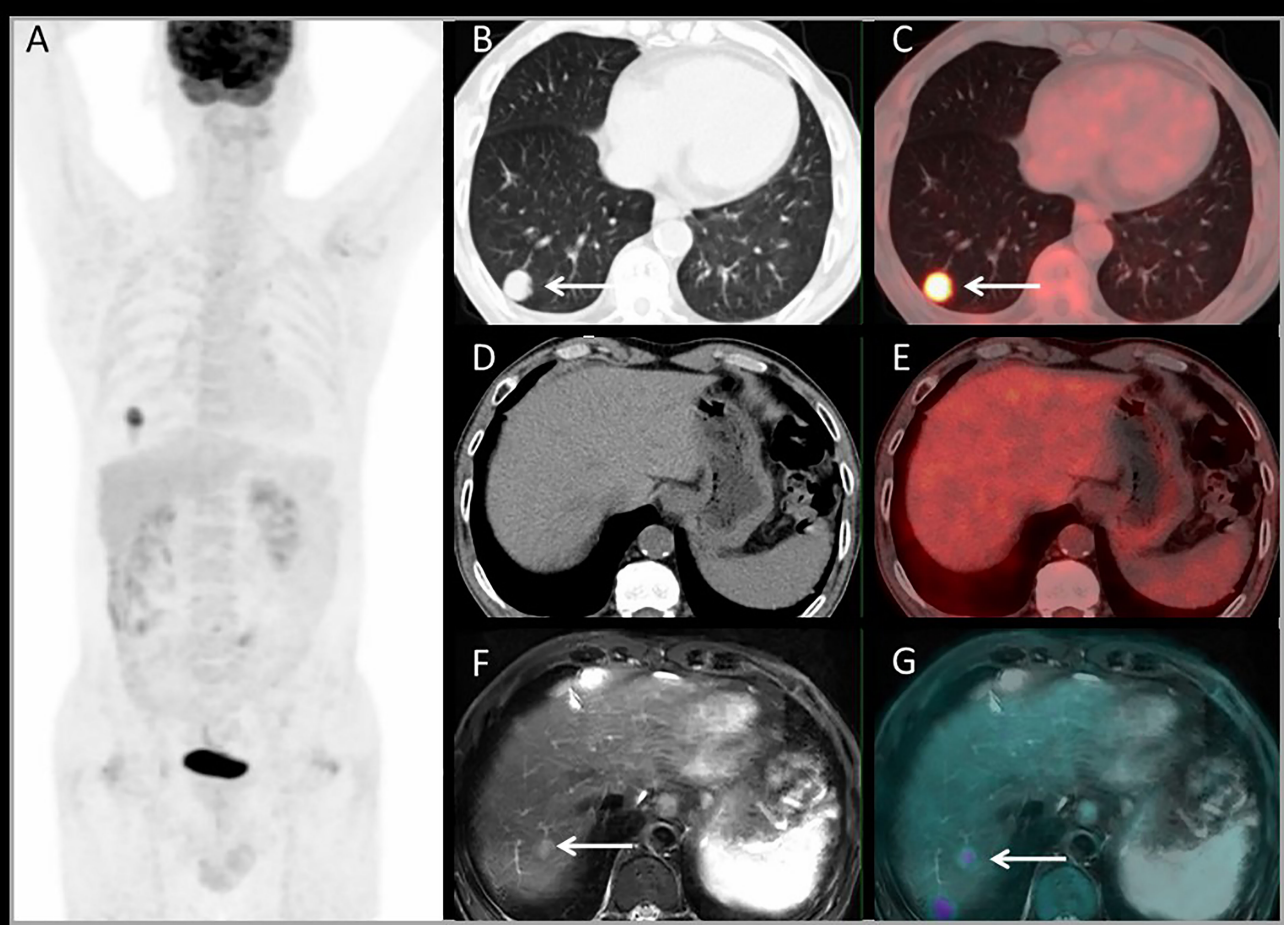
Images of a 63-year-old male with colonic liver metastasis. The MIP (A) of PET/CT showed a lesion on right lung but no ^18^F-FDG foci on liver. CT and PET/CT fusion images showed single lung metastasis with high ^18^F-FDG uptake (B, C) and no lesions were detected on liver (D, E). T2WI and PET/MRI fusion images showed a single lesion on right liver lobe with mild ^18^F-FDG uptake (F, G).
